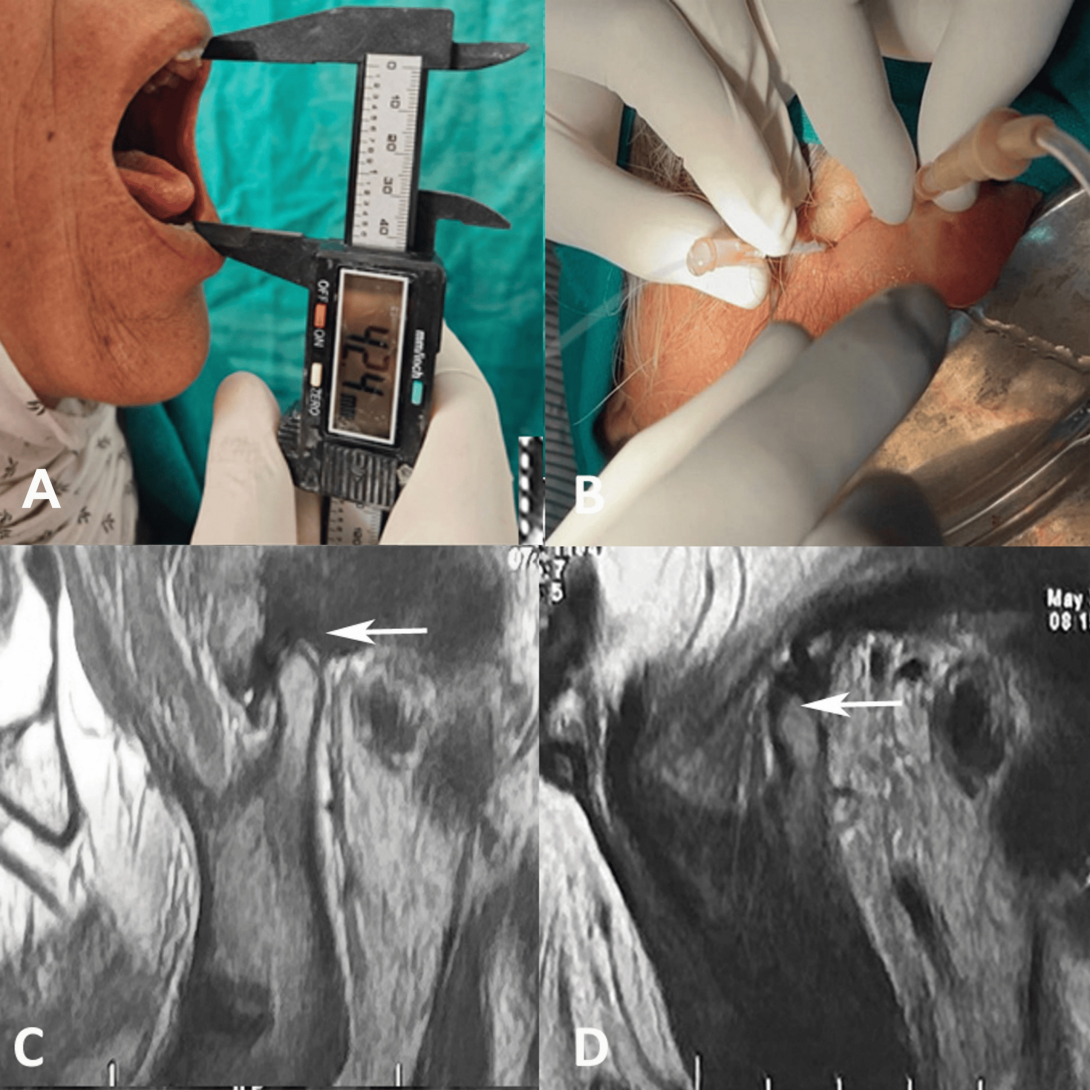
(A) Preoperative mouth opening (42.4 mm), (B) procedure of arthrocentesis of the right temporomandibular joint, (C) MRI scan with open mouth showing synovitis with effusion of the right temporomandibular joint, and (D) MRI scan with closed mouth showing synovitis with effusion of the right temporomandibular joint.
